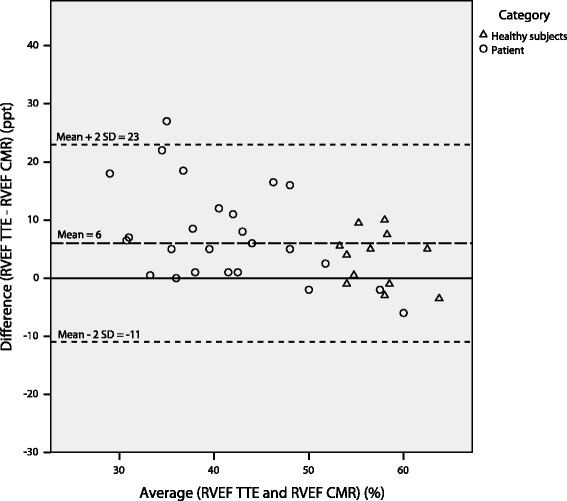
Bland-Altman plot of agreement between RVEF_TTE_ and RVEF_CMR._ Bland-Altman plot of the agreement between the mean values for RVEF calculated using the ellipsoid model on TTE measurements and RVEF derived from CMR. The dashed lines show the mean value and the limits of agreements. CMR = cardiac magnetic resonance, RVEF = right ventricular ejection fraction, TTE = transthoracic echocardiography
